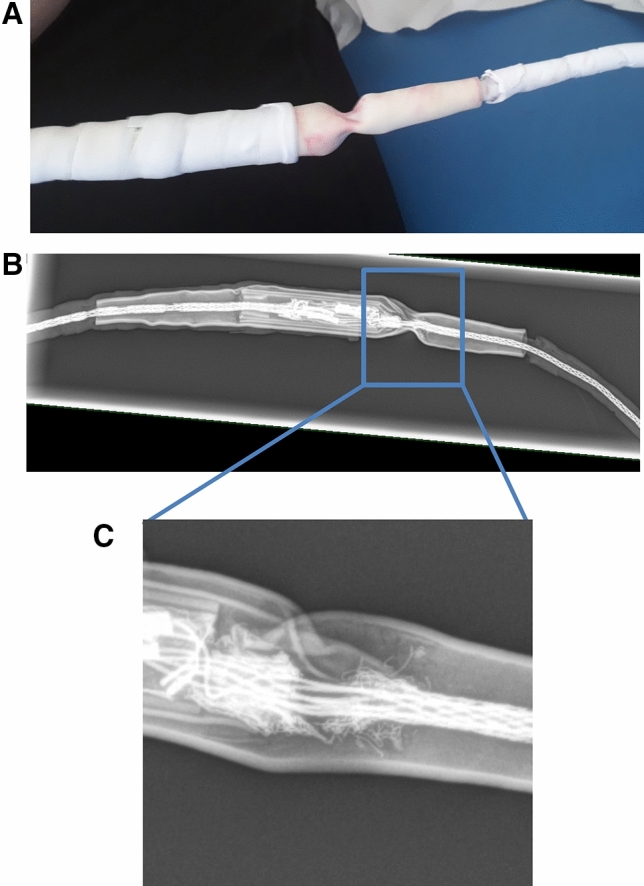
a Repaired driveline after removal of ResQ-tape with constriction, b X-ray of the constriction, c extension with cleft in the shield
